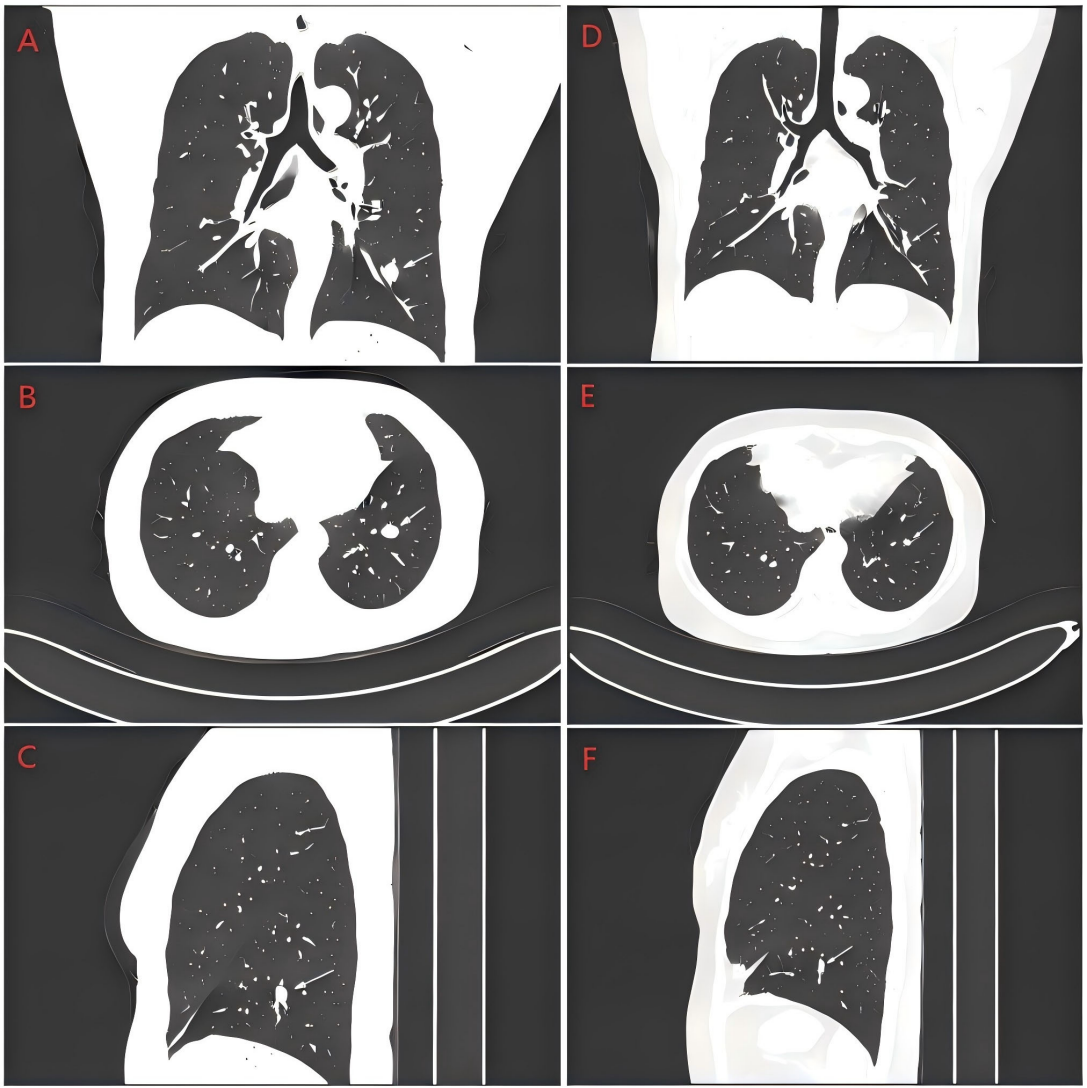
Lung CT images during recurrence and after treatment. (A) Lung CT images during recurrence (coronal plane); (B) Lung CT images during recurrence(transverse plane); (C) Lung CT images during recurrence(sagittal plane). (D) Lung CT images after treatment(coronal plane). (E) Lung CT images after treatment(transverse plane); (F) Lung CT images after treatment (sagittal plane). The white arrow indicates the lesion.
